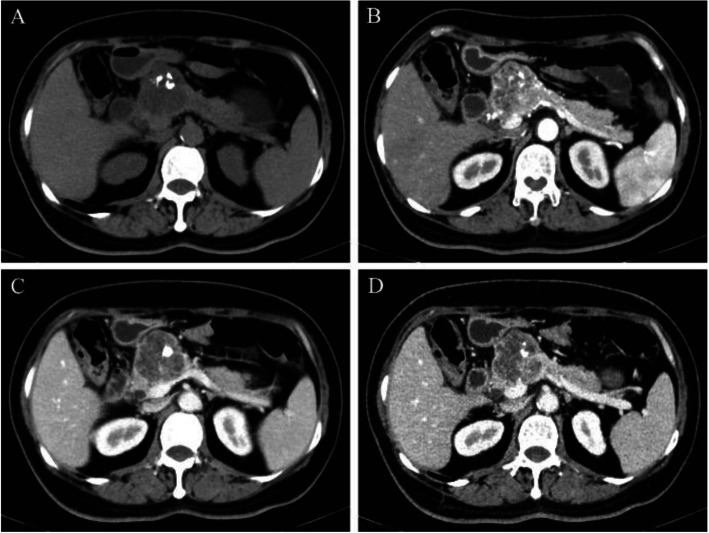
Dynamic CT scans of the pancreas showed a well-defined mass in the body of the pancreas. a Plain scan showed speckled and lamellar calcifications in the mass. b Arterial phase revealed mildly inhomogeneous enhancement. c Venous phase. d Delayed phase showed slight dilation of the main pancreatic duct
